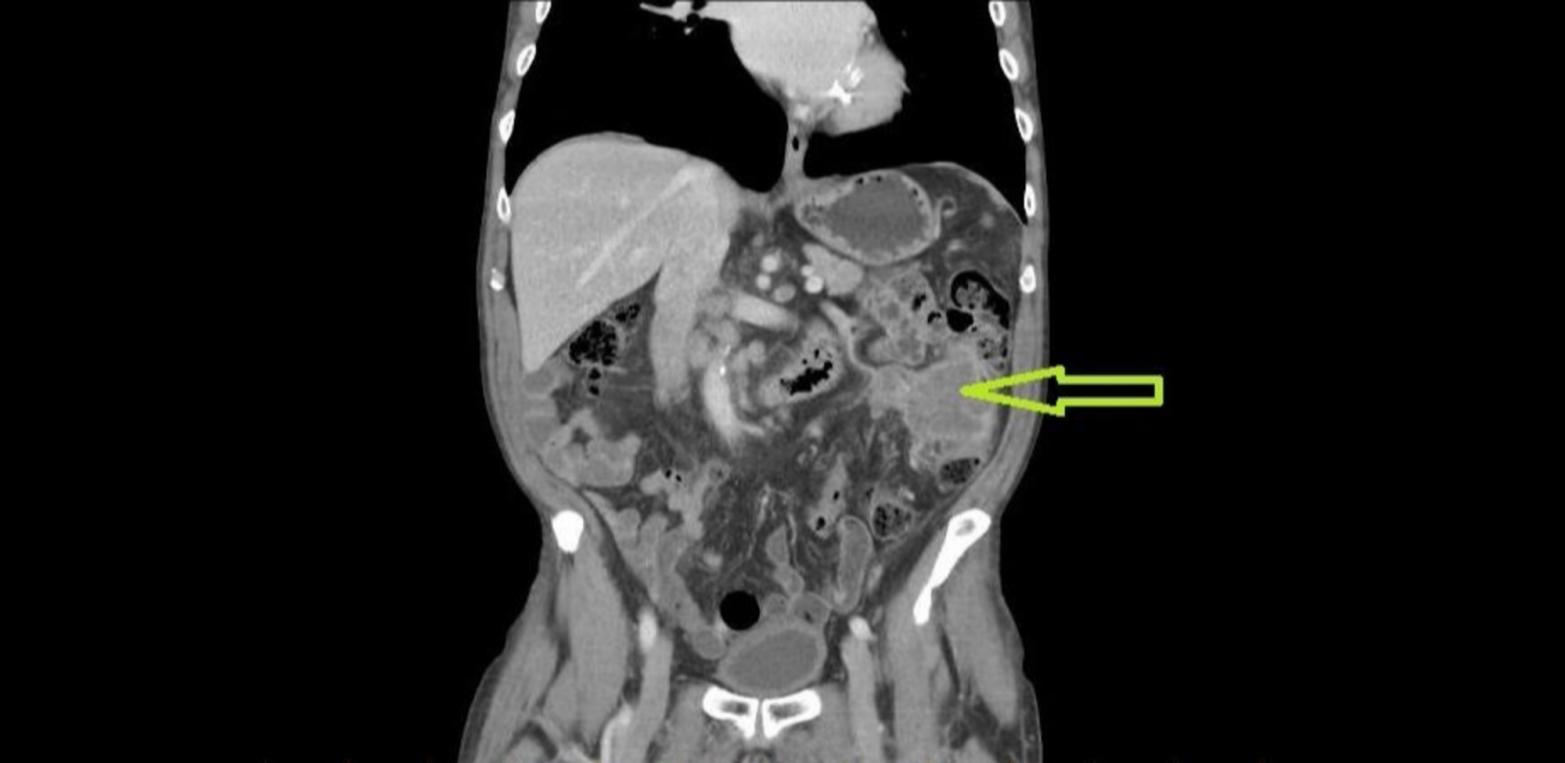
Coronal CT scan post-contrast revealing a colonic mass with medial extension into the mesentery.
